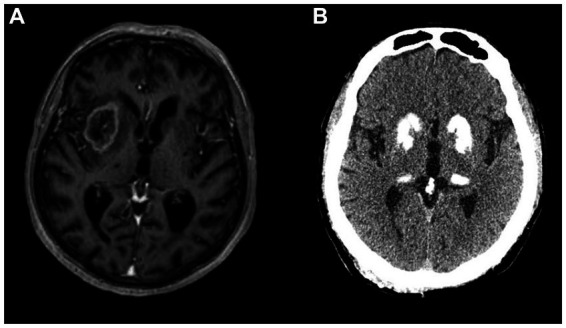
(A) Glioblastoma in right basal ganglia on gadolinium-enhanced T1-weighed MRI presenting with left hemiparkinsonism, gait instability and mild dysarthria. (B) Basal ganglia calcifications on CT scan in a patient with a disorder of calcium metabolism presenting with symmetric parkinsonism.
